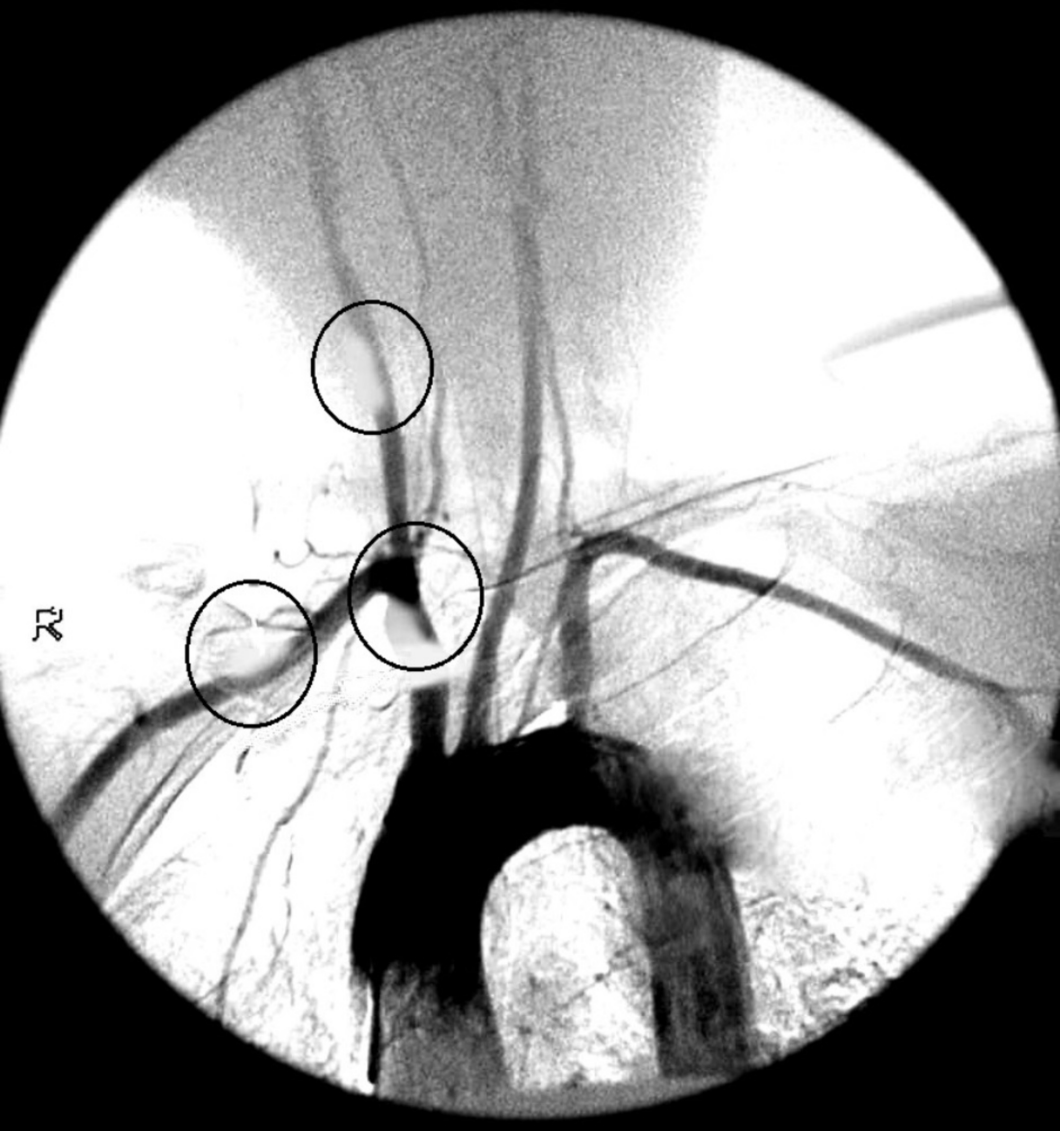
Computed tomography angiography showing occlusions stenoses.
